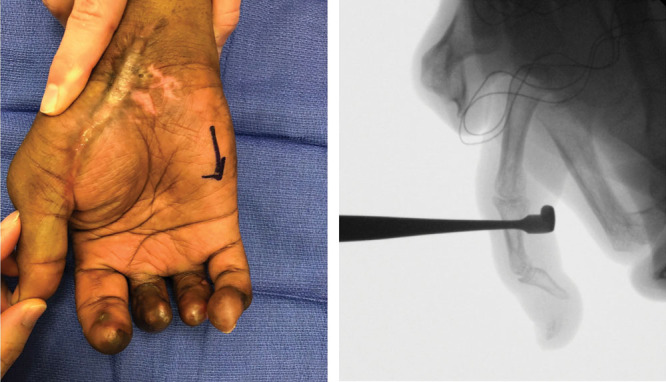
Preoperative appearance at the time of resuspension. A, Hand appearance 5 months after the original injury. B, Intraoperative x-ray demonstrating mobile volar pulp tissue.
